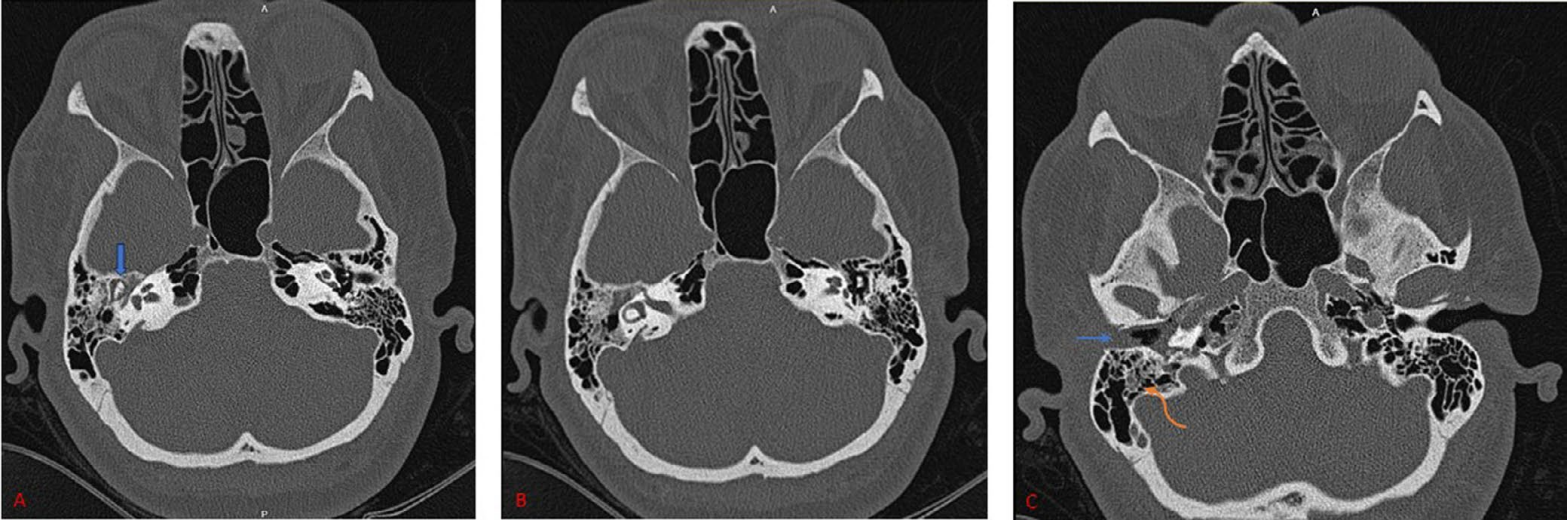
Axial temporal bone computed tomography (CT) scan. (A) Opacification in the attic (blue arrow). (B) Antrum is also opacified. (C) Few mastoid air cells have opacification (curve arrow), soft tissue density without any erosion in bony canal is seen (blue arrow), that was cerumen.
